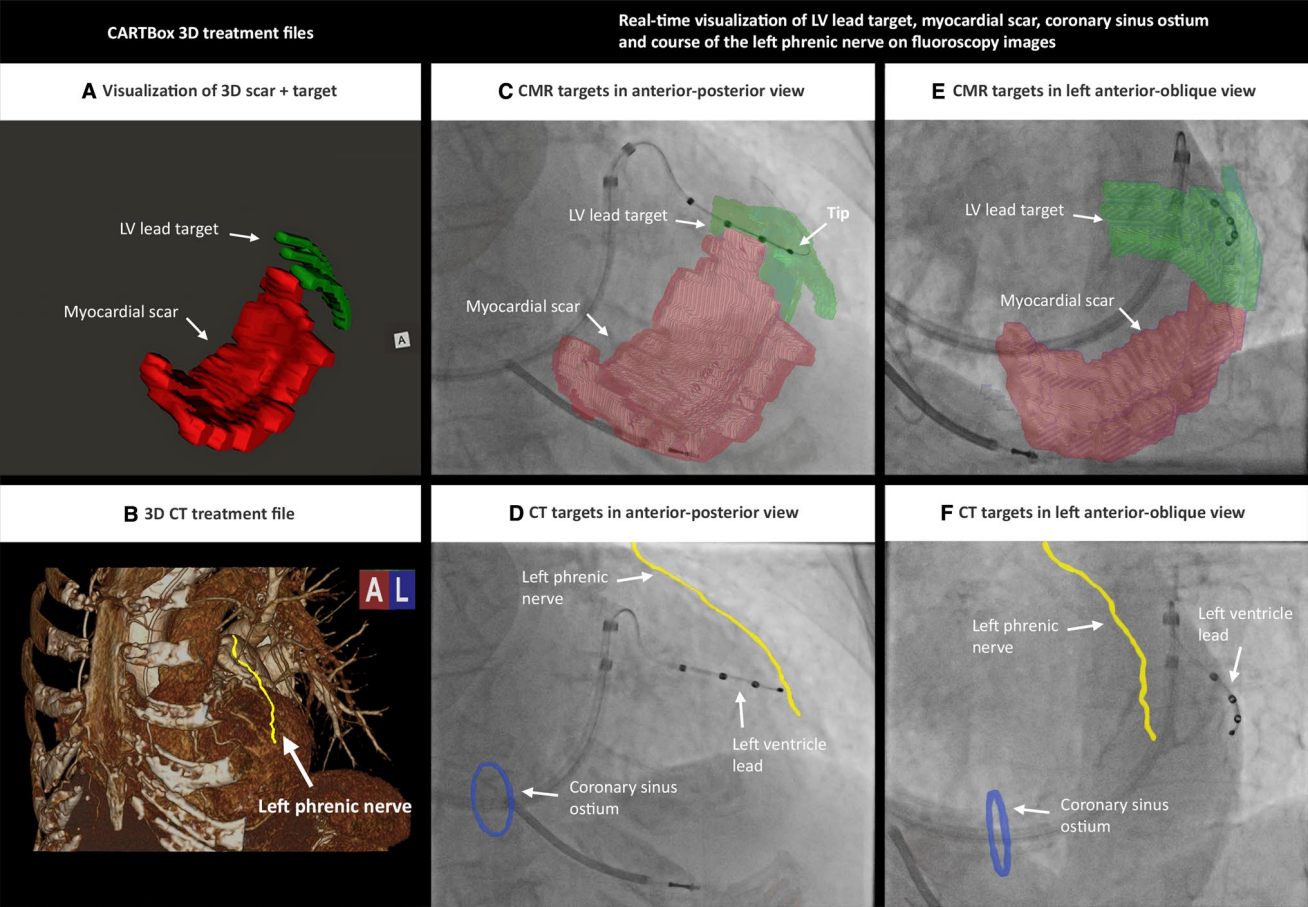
Real-time visualization of CMR and CT targets. a, b 3D-treatment file of CMR data (a) and CT data (b). c–f After 3D image fusion of the 3D-treatment dataset with fluoroscopy, the LV lead targets and scar segments (c, e) together with left phrenic nerve and coronary ostium (d, f) are visualized on live fluoroscopy during the LV lead implantation. *CMR* cardiac magnetic resonance, *CT* computed tomography
